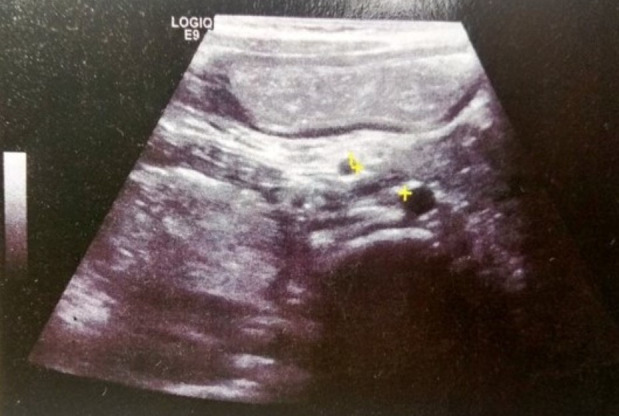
Abdominal Ultrasound proximal duo-denum with a reduced aorto–superior mesenteric artery (SMA) distance of 4 mm.
